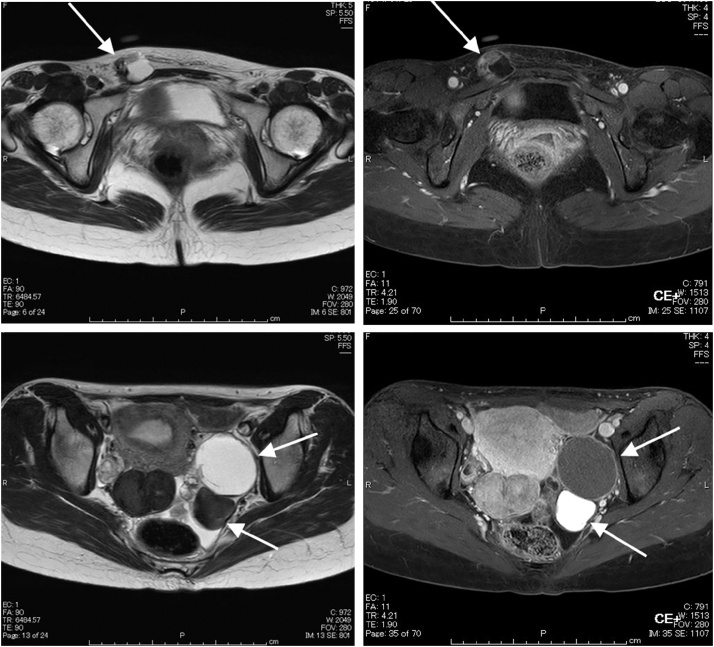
Preoperative MRI scans. Upper left: T2-weighted image, transverse plane. Right inguinal tumor (white arrow) enclosing the serous component; solid components are enclosed by a cystic wall with irregular mural hypertrophy. Upper right: T1-weighted image, fat suppression, transverse plane. Contrast enhancement of the hypertrophic areas of the cystic wall of the right inguinal tumor (white arrow). Lower left: T2-weighted image, transverse plane. Left ovarian tumor visualized as multilocular, with a high-signal intensity ventral chocolate cyst (white arrow, above) and a dorsal solid component (white arrow, below). Lower right: T1-weighted image, fat suppression, transverse plane. Contrast enhancement of the solid components of the dorsal cyst in the left ovarian tumor.
